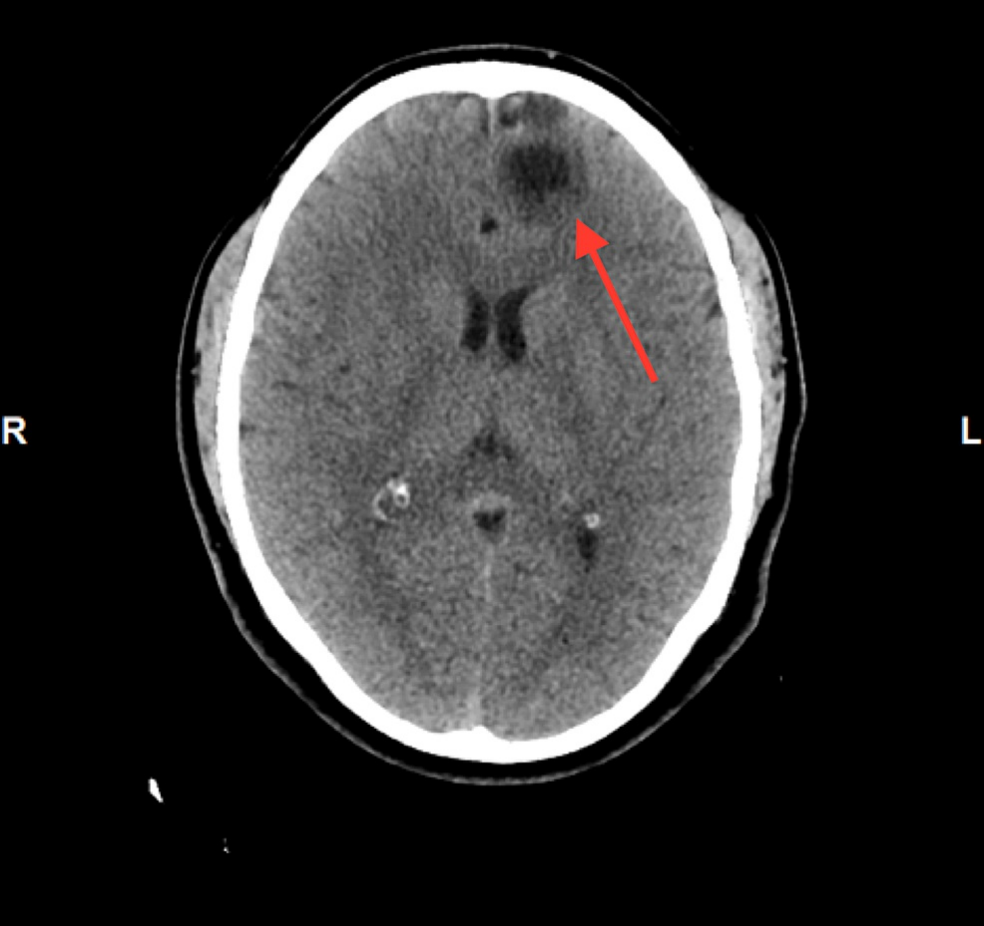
CT head showing a calcified cystic lesion in the anteromedial left frontal lobe CT: computed tomography
